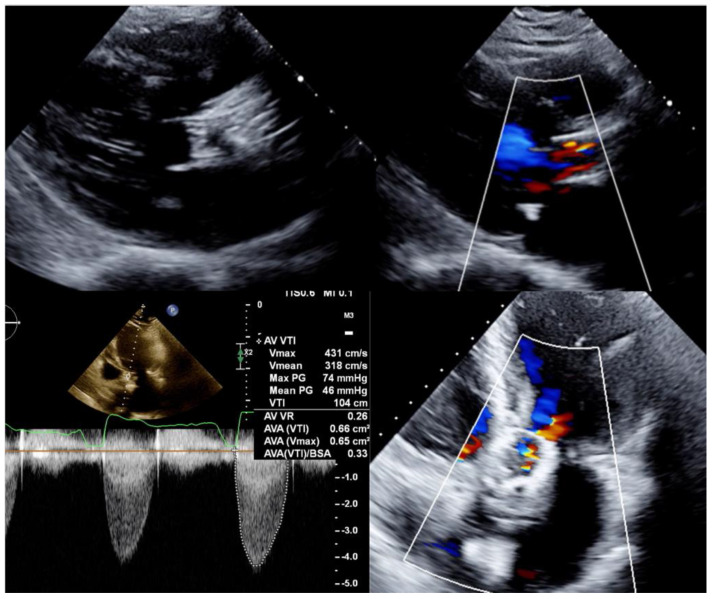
Transthoracic echocardiogram long-axis and 4-chamber views showing a degenerated CoreValve bioprosthesis with thickened leaflets, severe aortic stenosis, and moderate aortic regurgitation.
